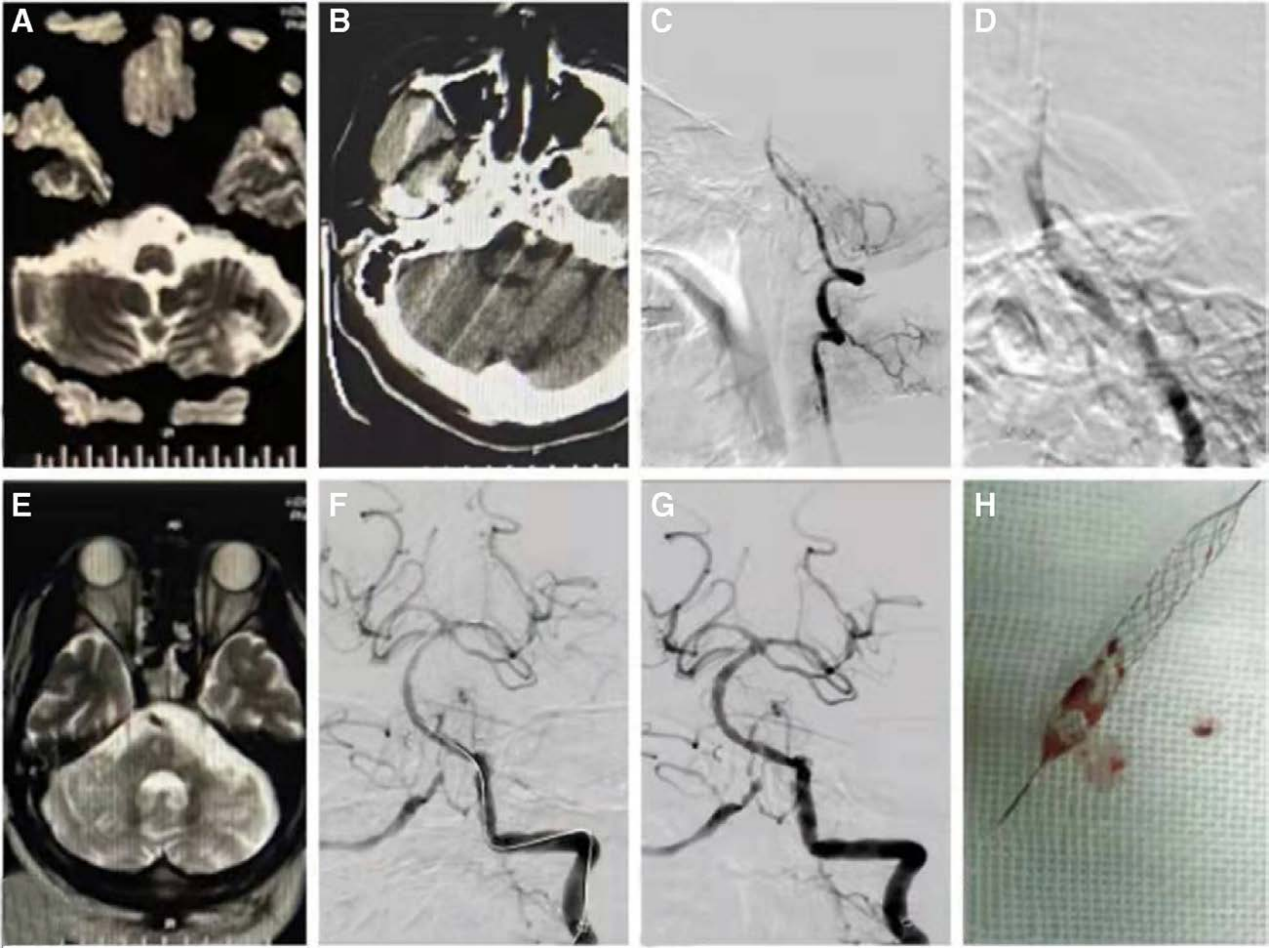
(A) DWI examination showed fresh cerebral infarction in bilateral cerebellar hemispheres and the right occipital lobe. (B) Brain CT showed hyperdense signs of the basilar artery. (C) Left vertebral artery lateral angiography showed basilar artery occlusion. (D) Lateral magnification showed that the thrombus was located in the basilar artery stent. (E) A week later, the abnormal signal of the right occipital lobe decreased. (F) The stent was sent through the blood vessel. (G) DSA showed basilar artery blood recovered TICI-3 flow. (H) The stent was withdrawn, and a thrombus was seen. CT = computed tomography.
